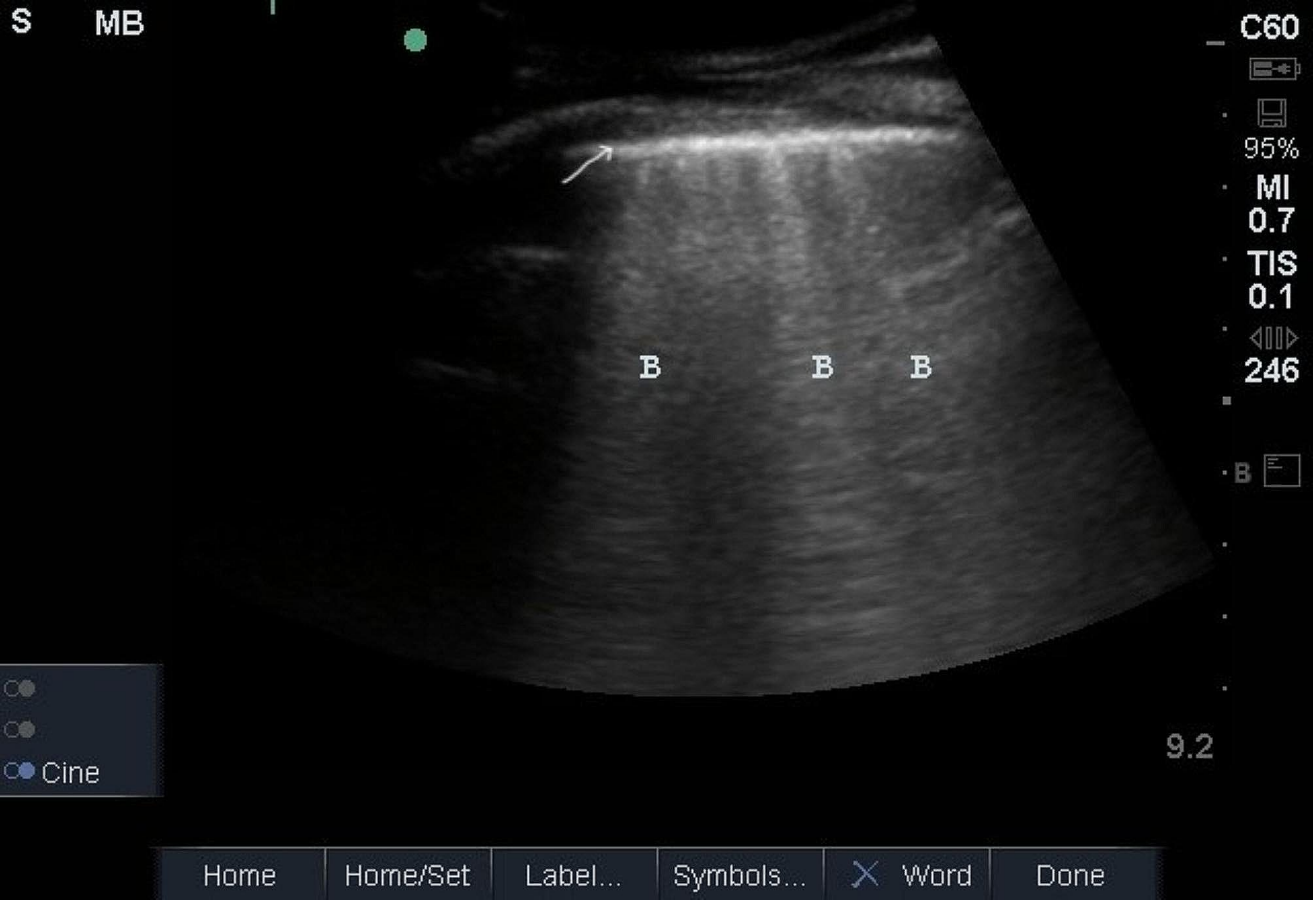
Example of a frozen loop of lung ultrasonography with three B-lines (B);, arrow showing the pleural line
